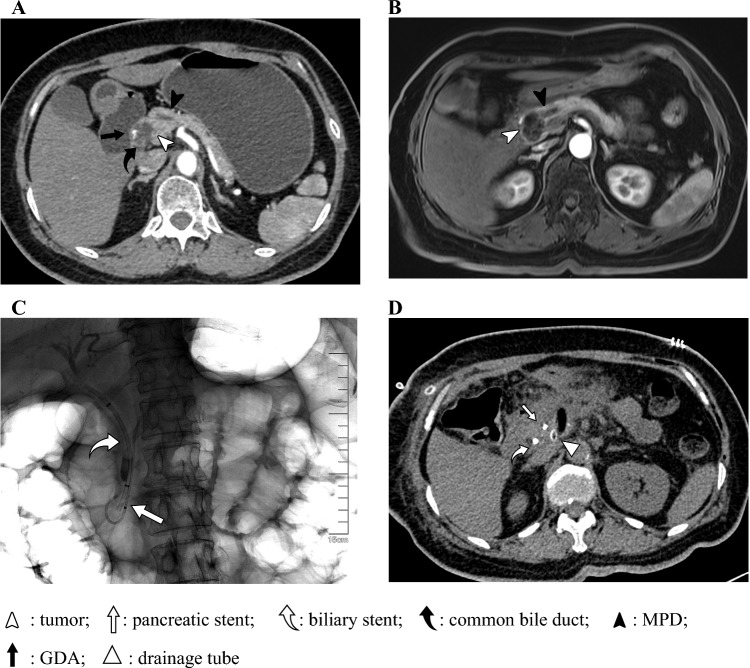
The patient had a 27 mm cystic lesion in the head of the pancreas. (a) Preoperative enhanced CT scan showed the tumor was in very close proximity to the GDA and the common bile duct. (b) Preoperative MRCP indicated mild dilation of the MPD, with the tumor potentially communicating with it. (c) Abdominal x-ray after biliary and pancreatic stent placement. (d) CT scan on POD 3 showed minimal intraperitoneal effusion. The blood amylase level was 87 U/L on POD 1, and postoperative pathology indicated a non-invasive IPMN with focal mild atypical epithelial hyperplasia. *GDA* gastroduodenal artery, *MPD* main pancreatic duct, *CT* computed tomography, *MRCP* magnetic resonance cholangiopancreatography, *POD* postoperative day, *IPMN* intraductal papillary mucinous neoplasm
